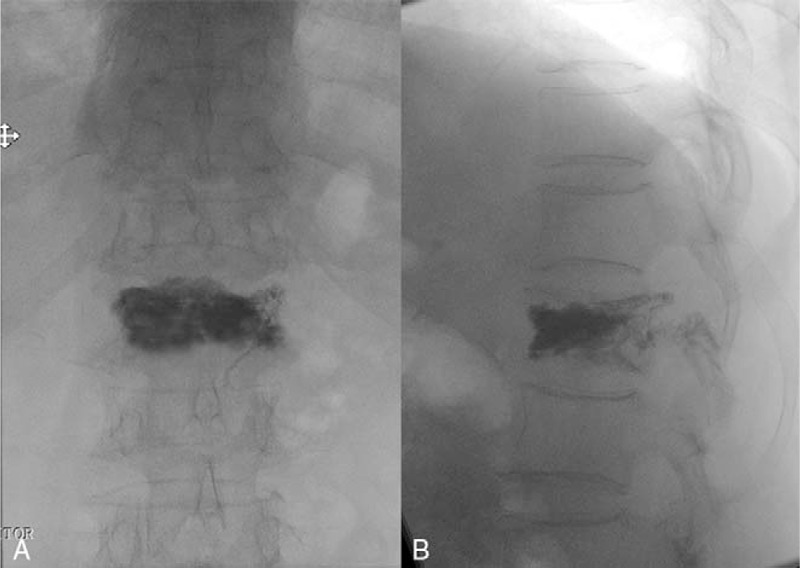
Postoperative X-rays of the lumbar. (A) Frontal and (B) lateral radiographs displayed good dispersion of the bone cement and recovery of the vertebral height.
